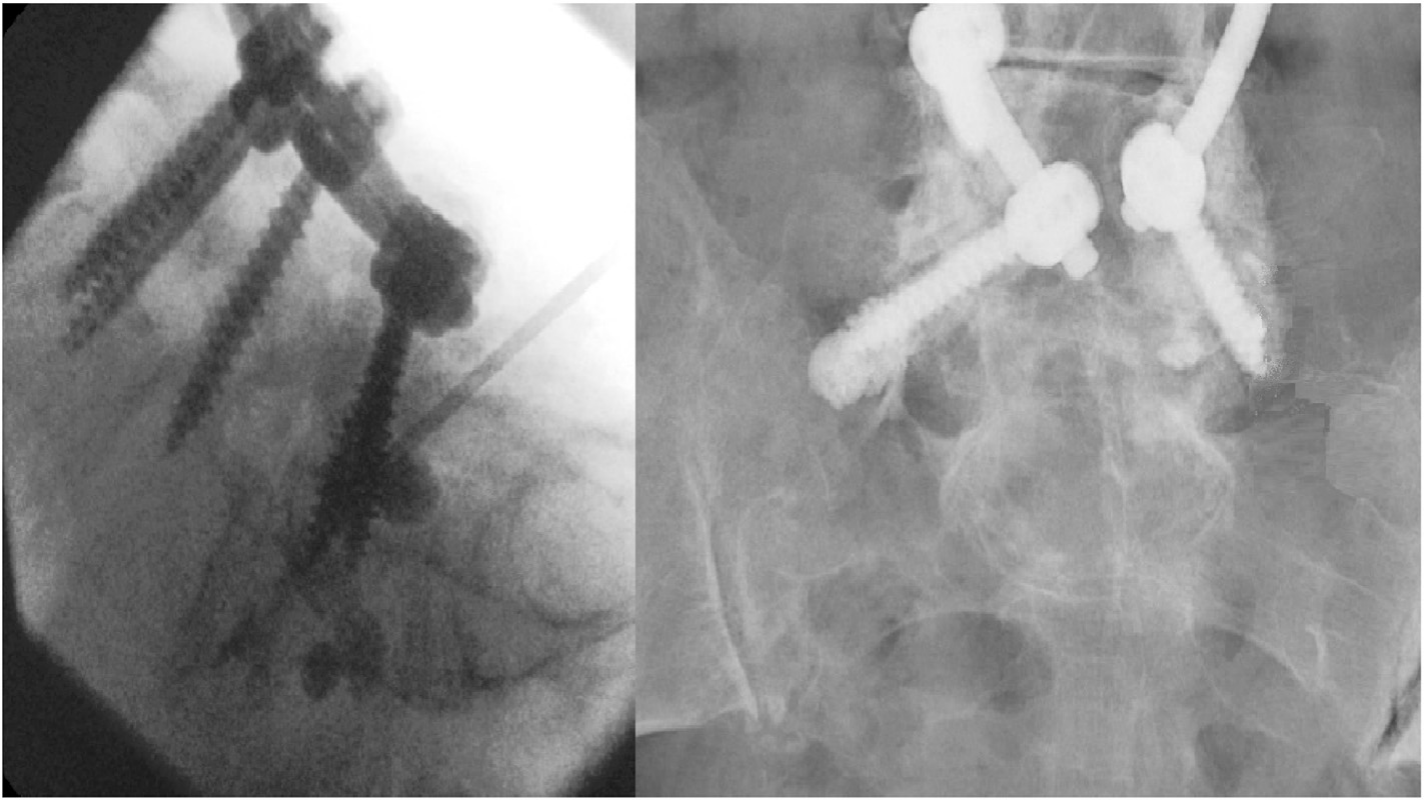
a & 2b: Lateral intra-operative fluoroscopy and postero-anterior post-operative radiography of salvage vertebral augmentation with PMMA around loose L5 screws (sacralized L5, previously revised with divergent trajectory, screw loosening post revision). Anterior cement leakage noted through anterior wall defect, no associated symptoms. Vacuum Disc Phenomenon noted at disc space indicative of persistent mobility.•Segmental stress-strain mismatch at the end of a long construct, with loss of vertebral integrity, usually PJF (Fig. 3a and b).
